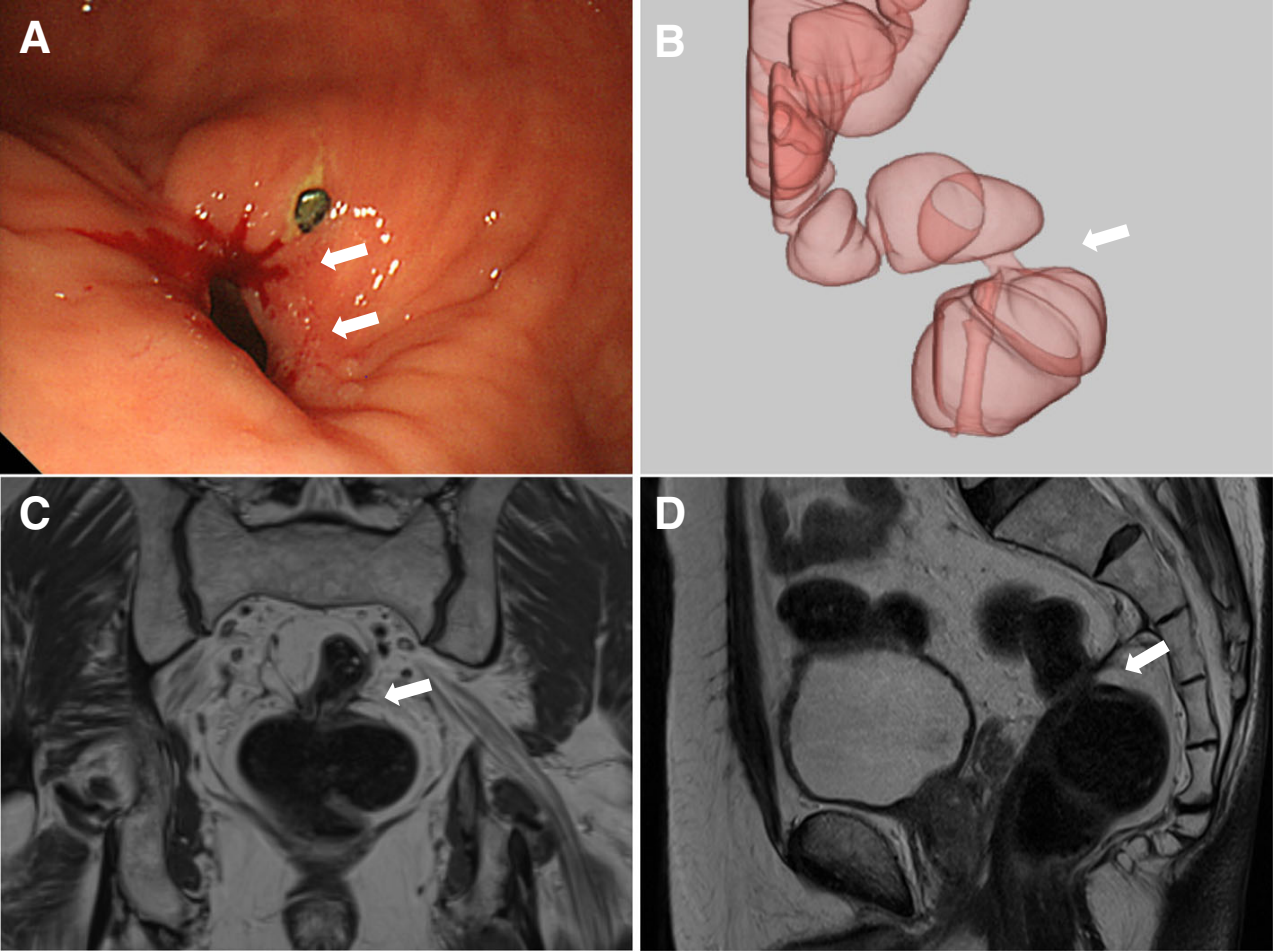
a Colonoscopy image showing a stricture in the lower rectum (white arrow). An endoscope of external diameter 9.9 mm could be passed, with resistance, through this stenosis. b Preoperative computed tomography–colonography image showing narrowing of the rectum at the stenosis (black arrow). c, d Magnetic resonance images showing rectal narrowing accompanied by muscular thickening (yellow arrow)
